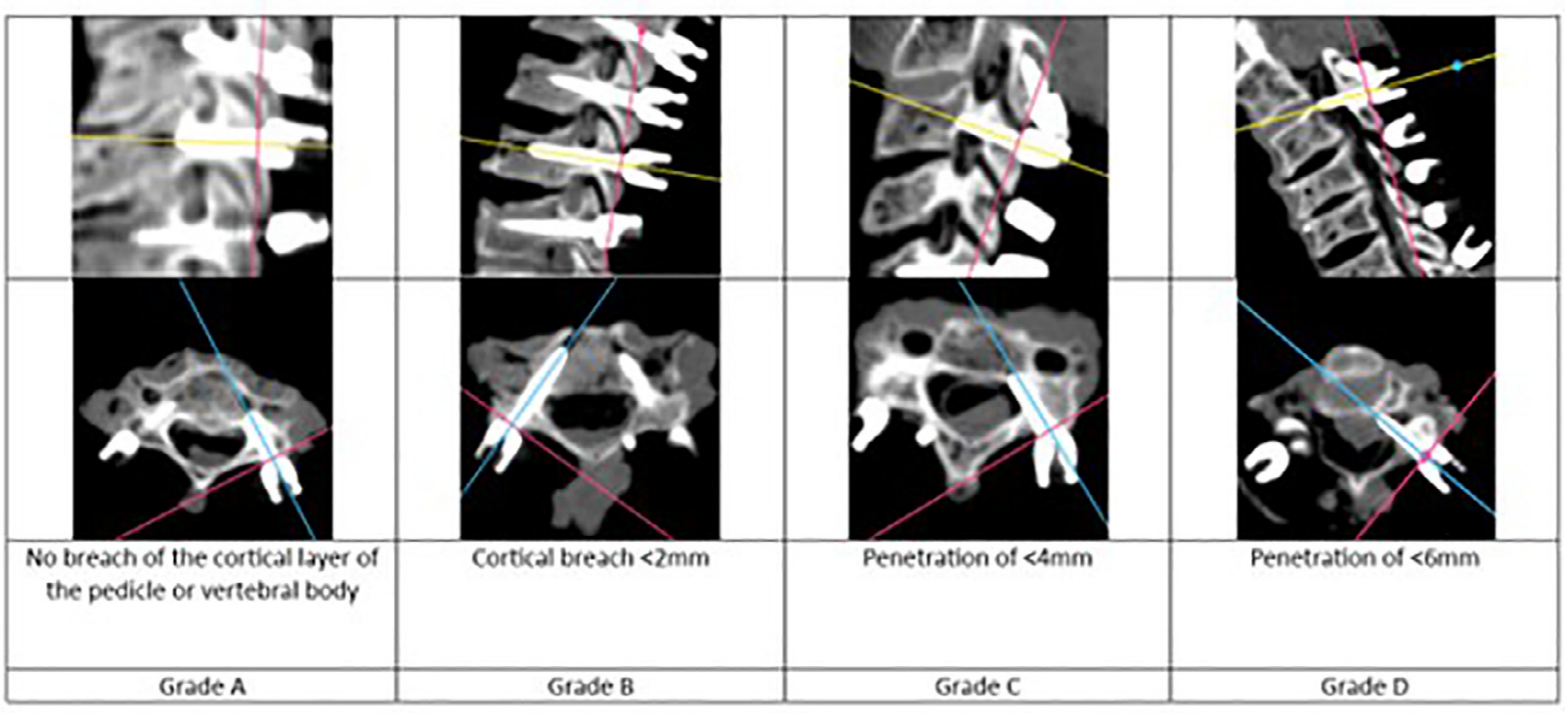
Representative axial and sagittal CT images of grades A-D Gertzbein- Robbins classification.
